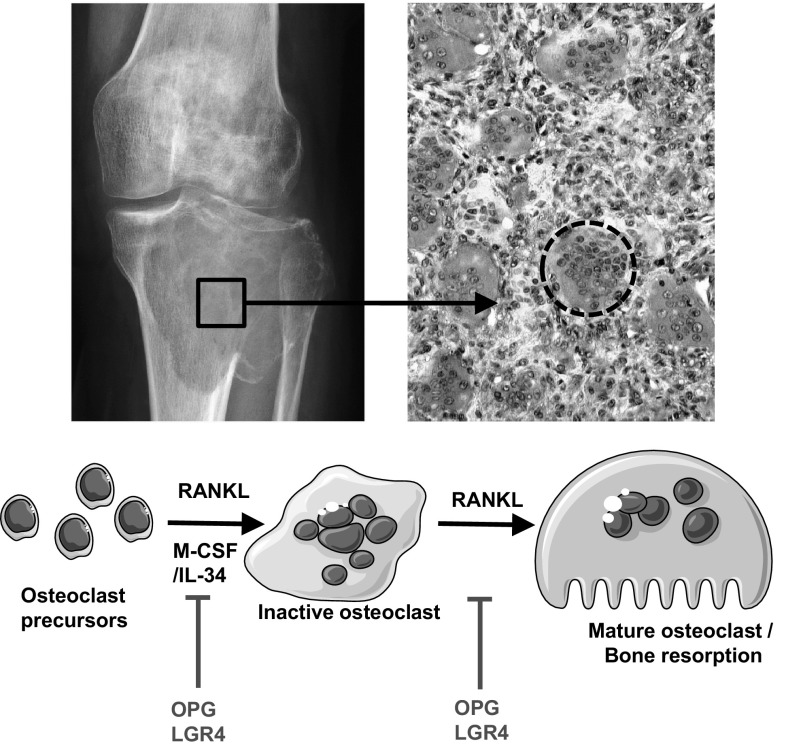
Giant cell tumours of bone: a benign entity with malignant features. Giant cell tumours of bone are composed of three main cell populations: stromal cells, macrophages and multinucleated osteoclast-like cells. These tumours are responsible for a marked local bone resorption leading to the formation of large osteolytic foci easily detectable by X-ray radiography. RANKL/M-CSF and/or RANKL/IL-34 released by stromal cell could induce the differentiation of macrophages considered as osteoclast precursors towards immature and mature osteoclasts resorbing bone. Soluble OPG and membrane LGR4 are two receptors that negatively control osteoclastogenesis. OPG acts as a decoy receptor to RANK resulting in blocked RANKL–RANK interactions. LGR4 is expressed by osteoclasts and binds to RANKL leading to Gαq/GS3K-β signalling and repression of the NFATc1 molecular pathway. *IL-34* Interleukin-34, *LGR-4* G-protein-coupled receptor 4, *M-CSF* Macrophage Colony-Stimulating Factor, *OPG* osteoprotegerin, *RANKL* Receptor of Nuclear factor kappaB Ligand
